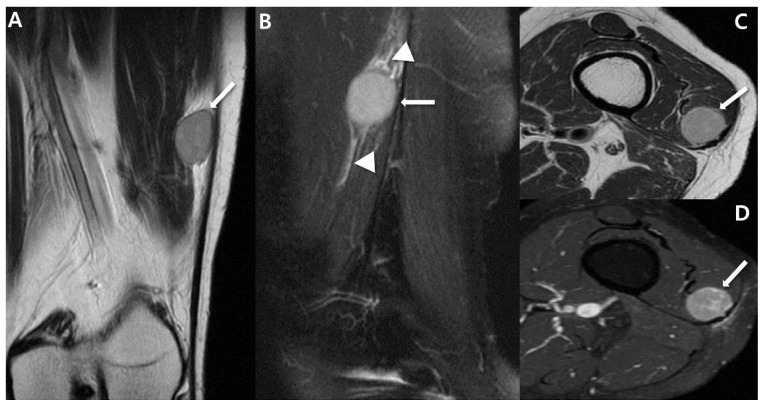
Non-aggressive features on MRI for small (<5 cm) synovial sarcoma of 42-year-old women (monophasic type): (A) Coronal T2-weighted image shows about 1.6 × 1.6 × 2.3 cm sized well-defined oval mass (arrow) with homogenous and relatively high signal intensity compared to adjacent muscle in the thigh. (B) Sagittal T2-weighted, fat-suppressed image shows a mass (arrow) abutting vastus lateralis muscle (arrowheads) with adjacent signal change. (C) Axial T2-weighted image shows well-defined oval mass (arrow) located in vastus lateralis muscle. (D) Axial T1-weighted post-contrast image shows relatively homogeneously enhancing mass (arrow).
